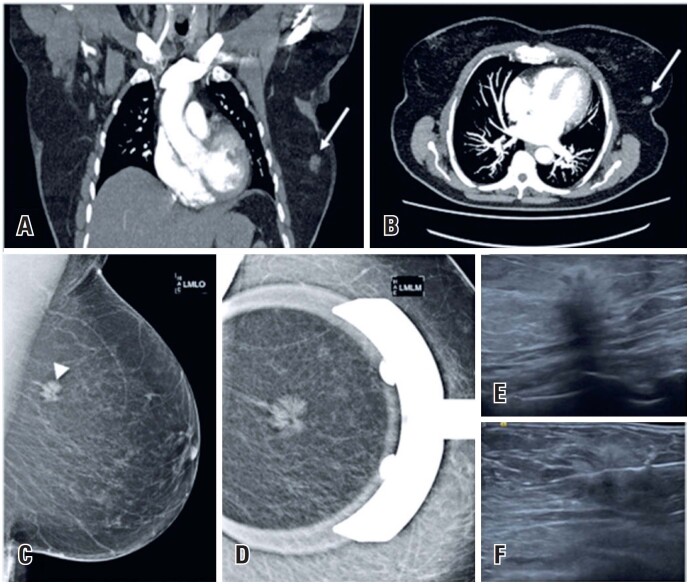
A 72-year-old woman arrived at to the emergency department with sudden shortness of breath and elevated serum D-dimer. Acute pulmonary embolism was suspected. (A and B) A chest computed tomography angiography (CTA) scan was requested and revealed no abnormalities, except for an incidental lesion on the left breast (arrows). (C and D) Mammography revealed an irregular spiculated mass - *BI-RADS 4* (arrowhead). (E and F) The lesion was identified on ultrasound and a US-guided core biopsy confirmed *invasive ductal carcinoma*
